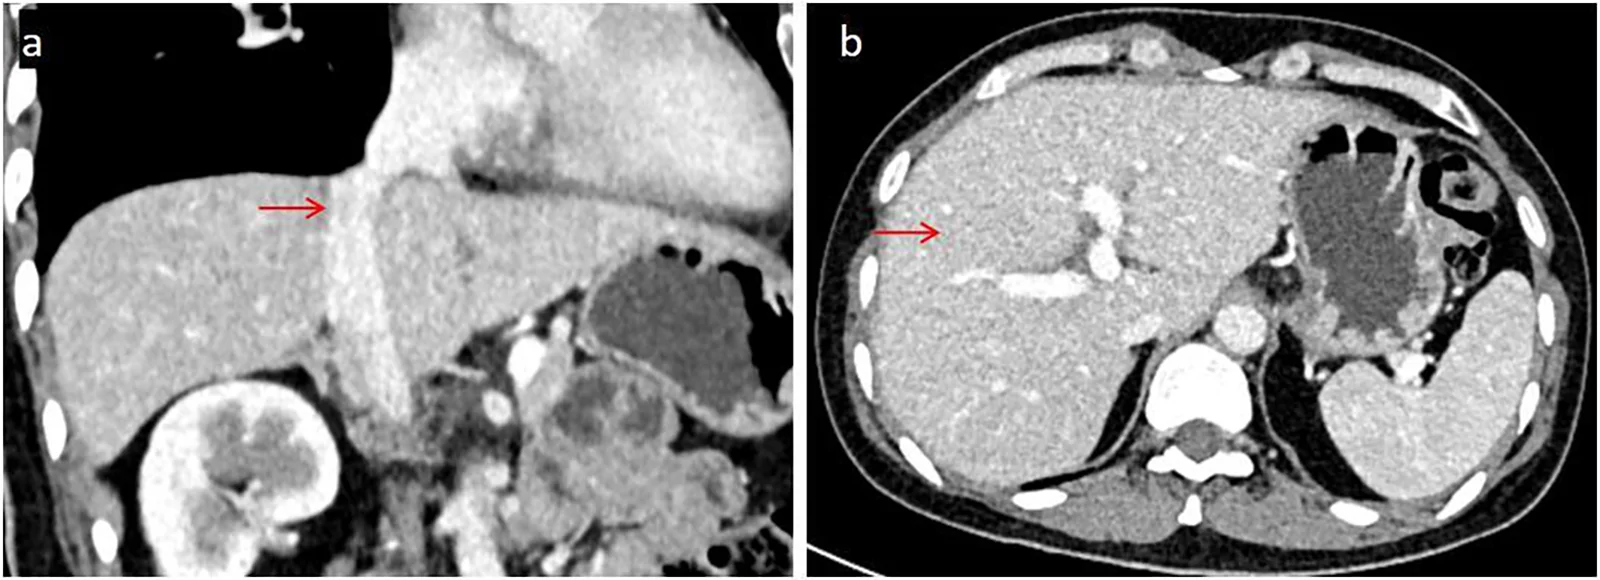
Follow-up upper abdominal contrast-enhanced CT at 6 months after discharge. (a) demonstrated the complete resolution of the IVC bacterial embolus; (b) demonstrated the complete resolution of the hepatic abscess and hepatic ischemic infarct lesions.
